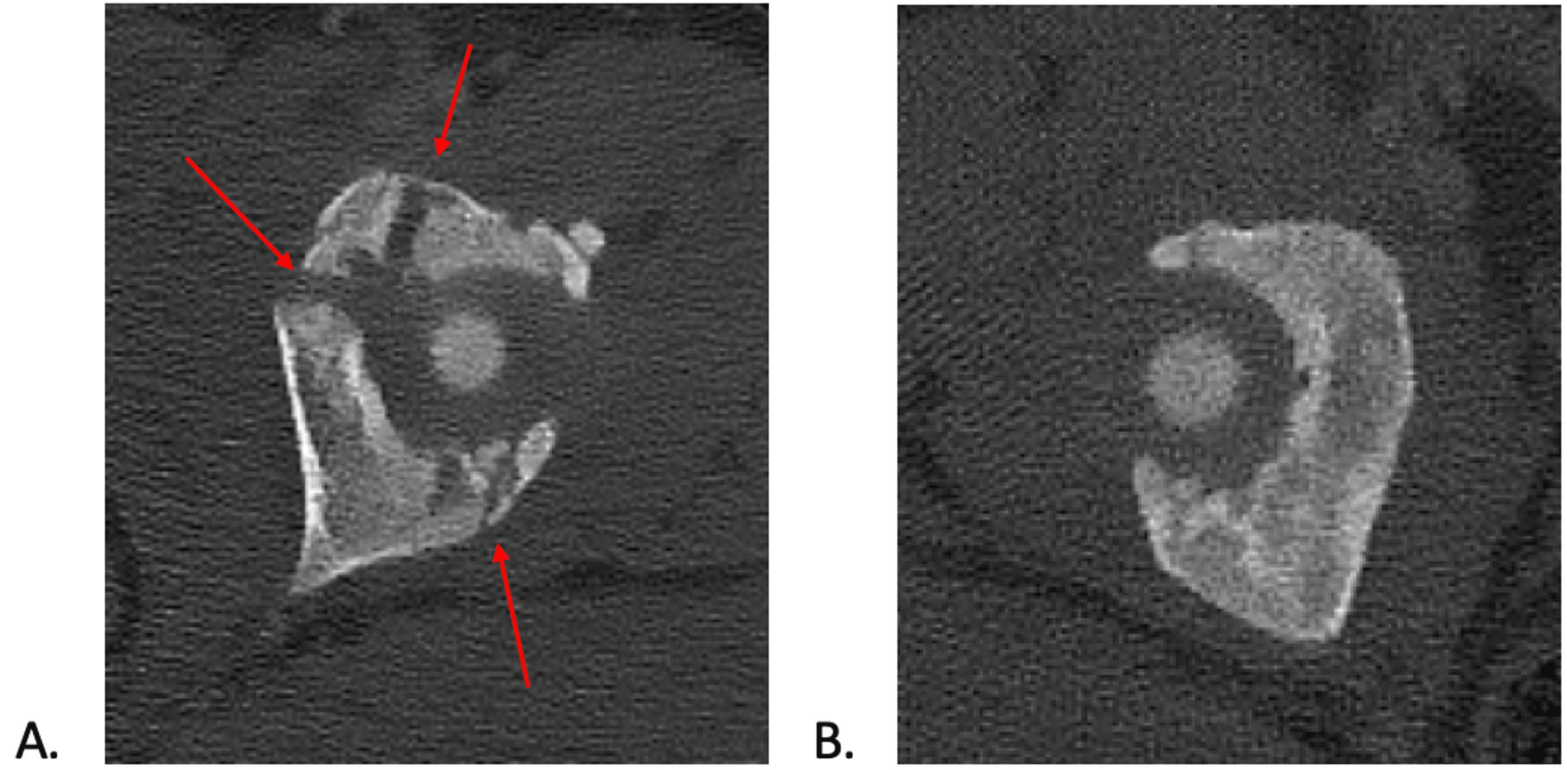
Select axial cuts from computed tomography scan of (a) left triradiate cartilage acetabular fracture and (b) uninjured acetabulum comparison.
